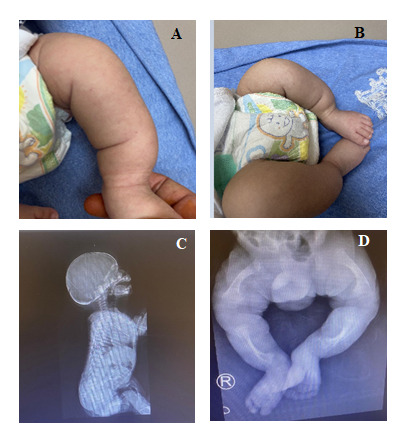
Clinical features of the patient with TMEM38B related phenotype A. and B. Images of the lower extremities showing mild shortening and bowing. Bilateral talipes equinovarus deformity was observed; C. and D. Representative radiograms of a patient with *TMEM38B *at 8 months of age. Skull radiographs showing generalized osteopenia, thin cortex of calvarium, and thickening of the occipital part of the skull. Patent sutures with no evidence of Wormian bone. Lateral spine view of the whole body showing generalized osteopenia and normal shape and height of vertebrae with maintained disc space. Chest showing osteopenia, thin ribs, mild widening of the costochondral junctions of the dorsal ribs and decreased aeration of both lungs. Right upper limb radiograph showing opteopenia. Anteroposterior view of lower limbs showing significant bilateral bowing and femoral deformity. The bowing was more prominent in the lower extremities in a background of metaphyseal irregularity. Diffuse osteopenia and healed fracture of the distal left femoral metaphysis were noted.
